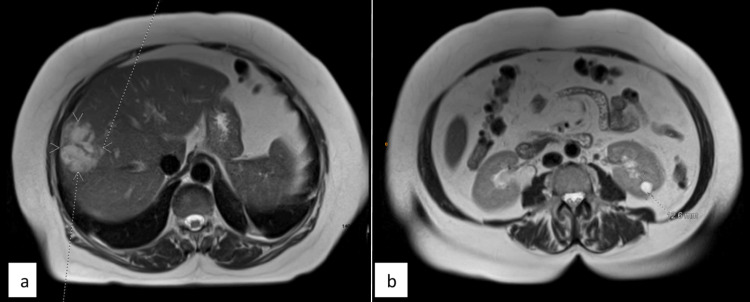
(a) An axial view of the T2-weighted image of the MRI abdomen and pelvis demonstrates a multiloculated right hepatic lobe lesion accompanied by surrounding reactive hyperemia, suggesting a liver abscess. (b) An axial view of the T2-weighted image of the MRI abdomen and pelvis reveals an obstructing calculus in the right mid ureter, leading to moderate upstream hydroureteronephrosis. In Figure 3a, the dotted arrowheads in white color indicate the multiloculated right hepatic abscess, while the dotted lines in the background are artifacts.
